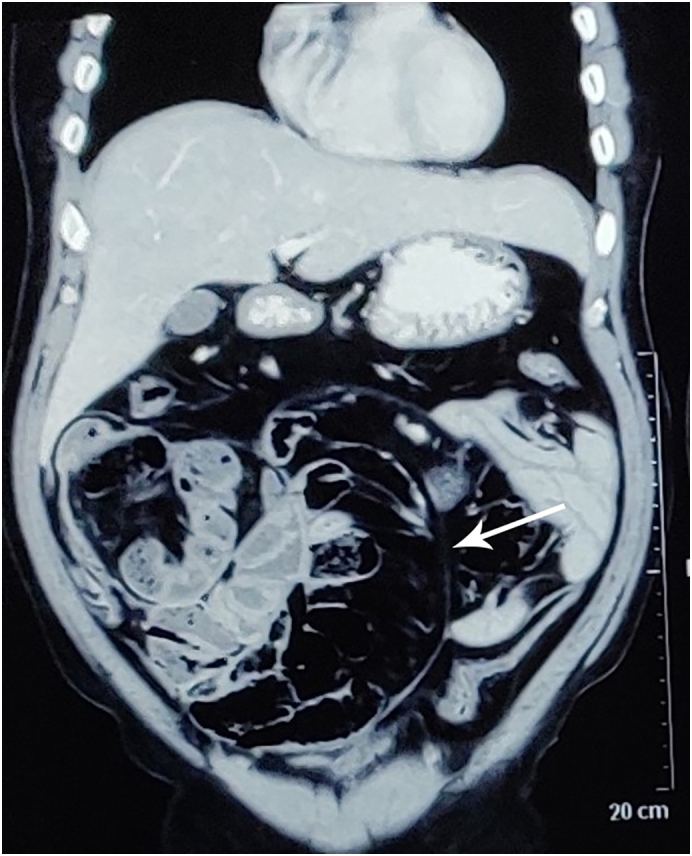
CT image showing the cocoon membrane encasing the bowel loops (arrow).
